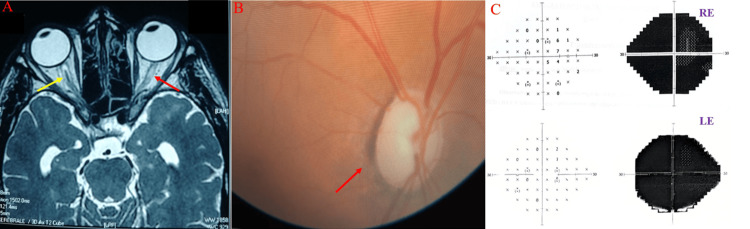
(A) MRI of the brain (T2 sagittal section) showing right optic atrophy with enlargement of the optic nerve sheath (yellow arrow) and hyperintense signals in the right optic nerve (red arrow). (B) Fundus examination showing cupping and pallor of the optic disc (red arrow). (C) Humphrey visual field test showing total vision loss in both eyes.
